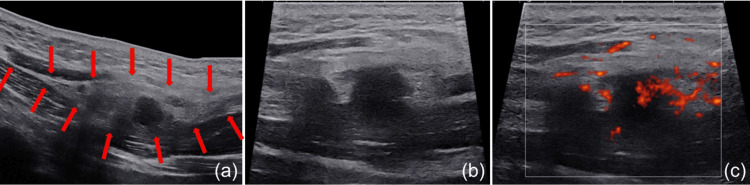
First ultrasound findings in our hospital on March 1, 2023 The superficial fascia of the left levator scapulae muscle was thickened, appearing as a fusiform mass with a scope of approximately 5.9 × 1.7 cm. A low echo area was visible at its center, with unclear boundaries, and the edges of the mass extended along the fascia. Ultrasound power Doppler suggested that the lesion had relatively rich blood flow signals. The ultrasound examination reported that the lesion was possibly nodular fasciitis. (a, panoramic ultrasound imaging of the lesion; b, extended field-of-view ultrasound imaging of the lesion, B-mode ultrasound; c, extended field-of-view ultrasound imaging of the lesion, power Doppler imaging)
